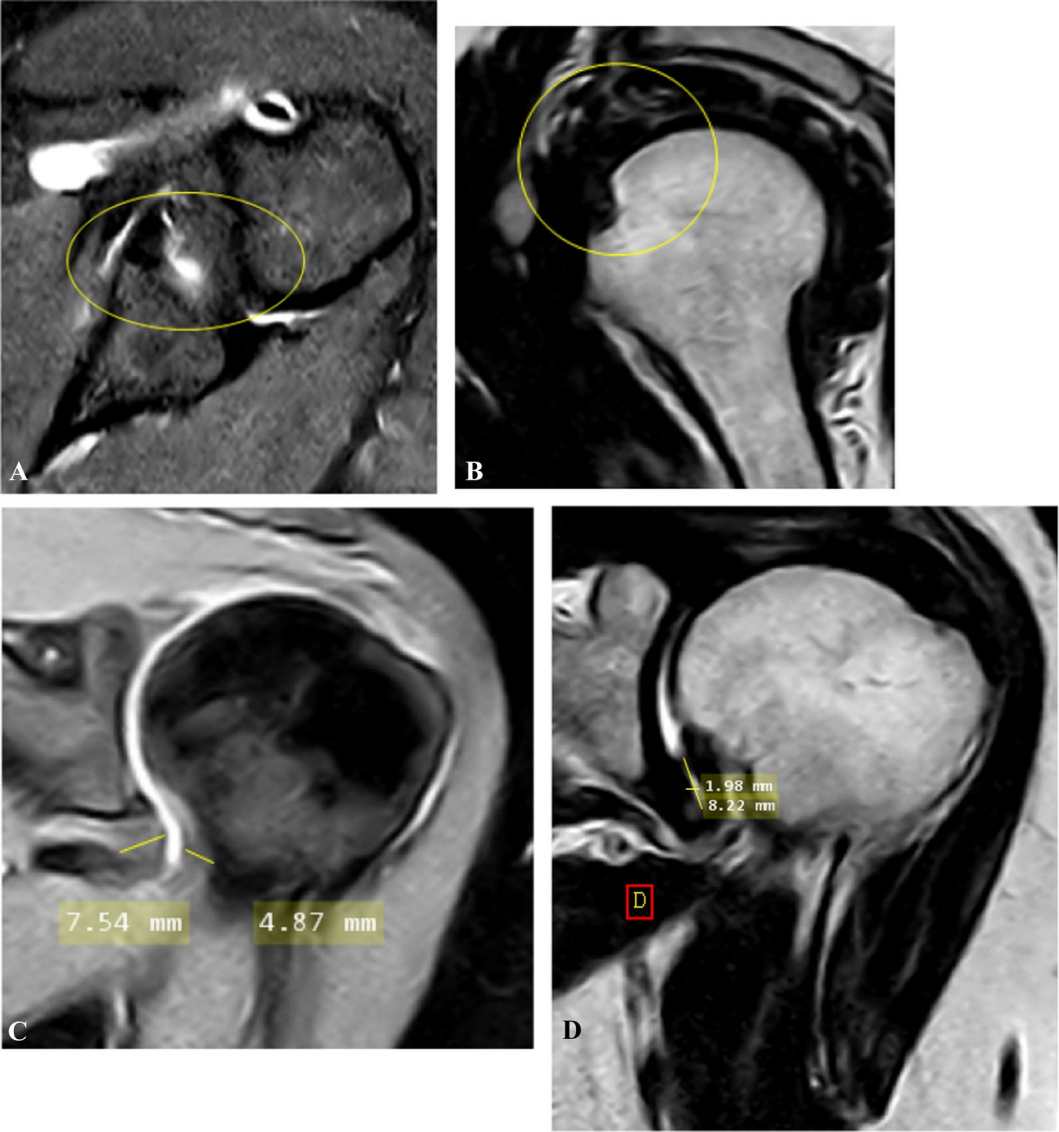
A Oblique axial fat-suppressed PD-weightrd MR image shows anterior and posterior extracapsular edema (hyperintensity) (encircled area). B Oblique sagittal T2-weighted MR image shows partial obliteration of subcoracoid fat triangle (encircled area). C Oblique coronal fat-suppressed PD-weighted MR image shows measurement of thickest portion of joint capsule in humeral and glenoid portions of axillary recess. D Oblique coronal T2-weighted MR image shows measurement of maximal height and width of axillary recess
